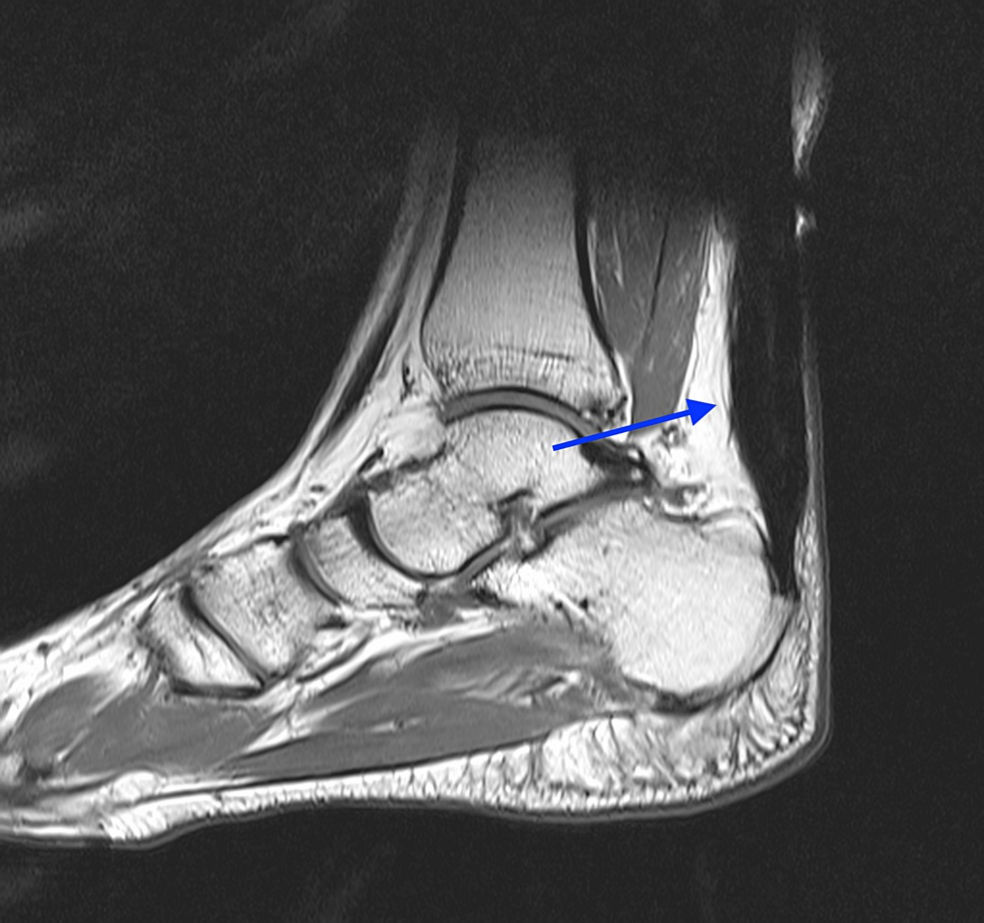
MRI of the left foot: A T1 weighted image depicting complete healing of the repair (blue arrow)
